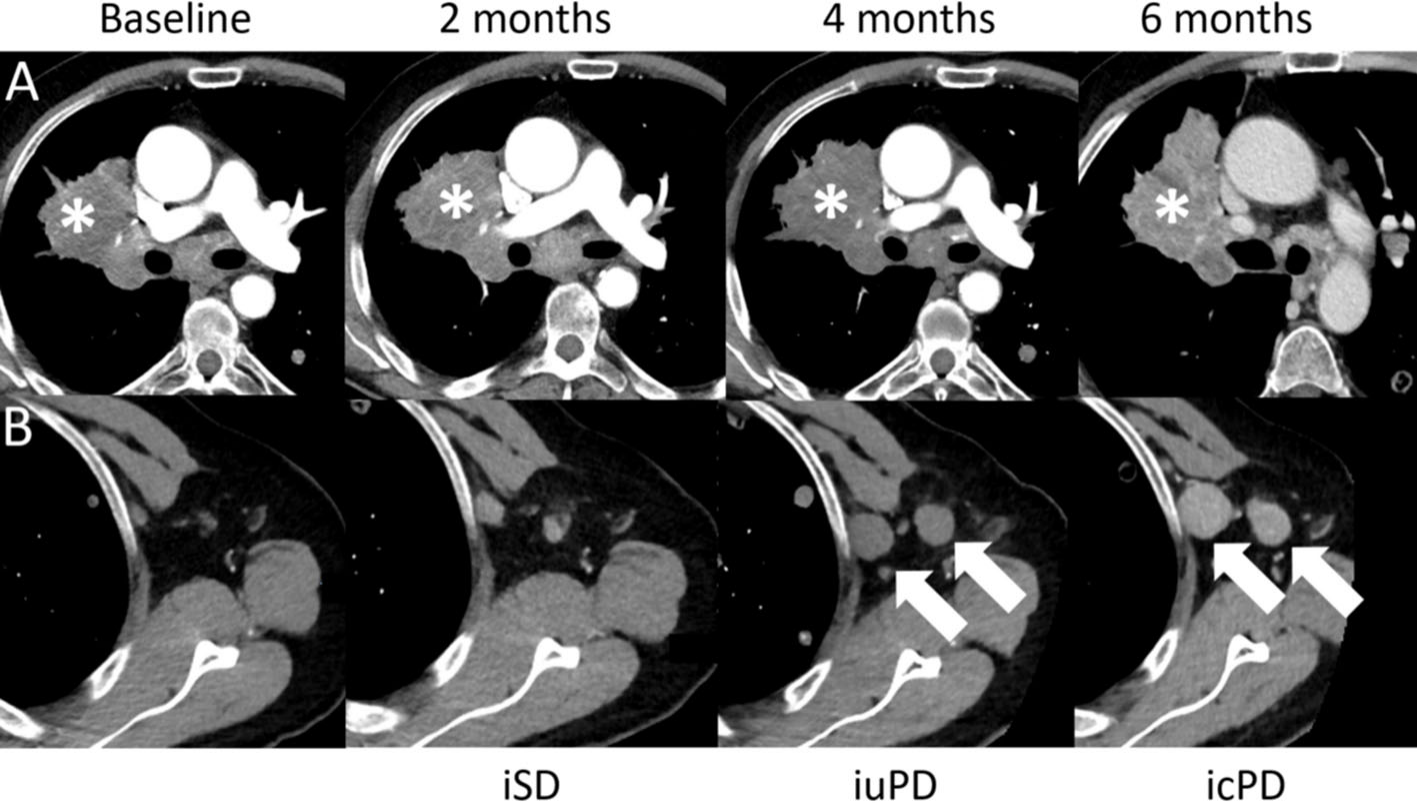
Confirmed progressive disease (icPD) in a 64-year-old male with right hilum adenocarcinoma treated with nivolumab (anti-PD-1) and anti-Lymphocyte Activation Gene-3 (LAG-3). Baseline contrast-enhanced CT demonstrates the right hilum mass (A,*). The 2-months follow-up showed stable disease (iSD). However, at the 4-months follow-up, the right hilum mass grew (A,*) and enlarged axillar lymph nodes appeared [(B), arrows], consistent with unconfirmed PD (iuPD). At the 6-months follow-up, confirmed PD (icPD) was established with continued growth of the right hilum mass (A,*) and the axillar lymph nodes [(B), arrows].
